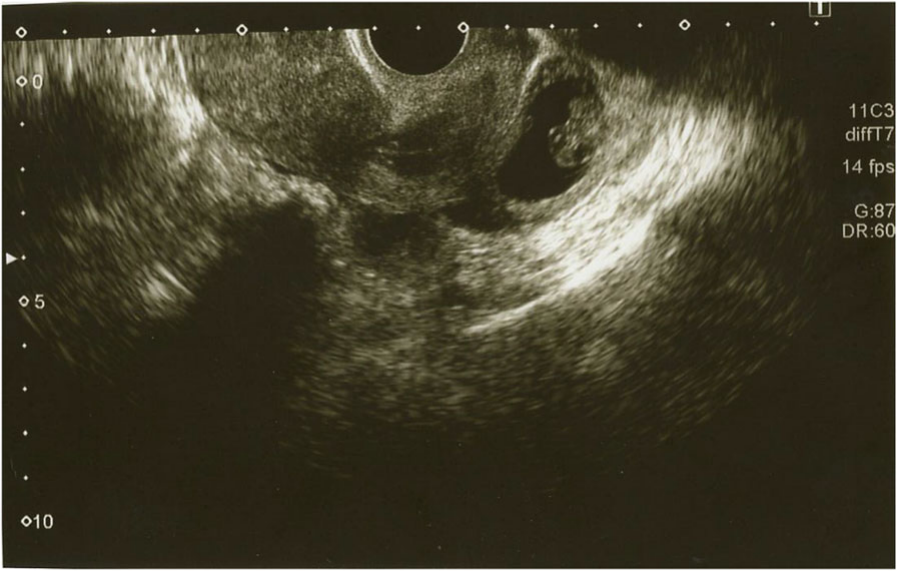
Ultrasound of gestational sac near Douglas cavum
